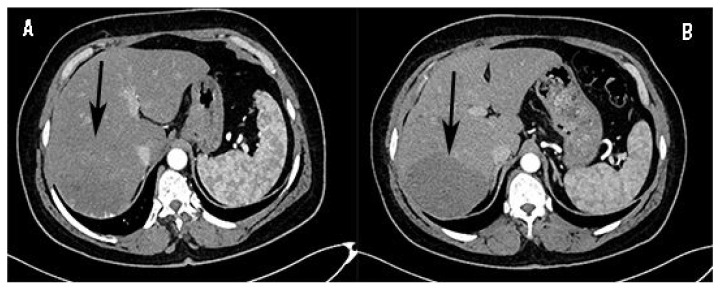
A: Arterial phase CT image of a hepatic giant hemangioma before TAE treatment arrow shows the lesion. B: Arterial phase CT image of a hepatic giant hemangioma 12 months after TAE treatment arrow shows the lesion.
